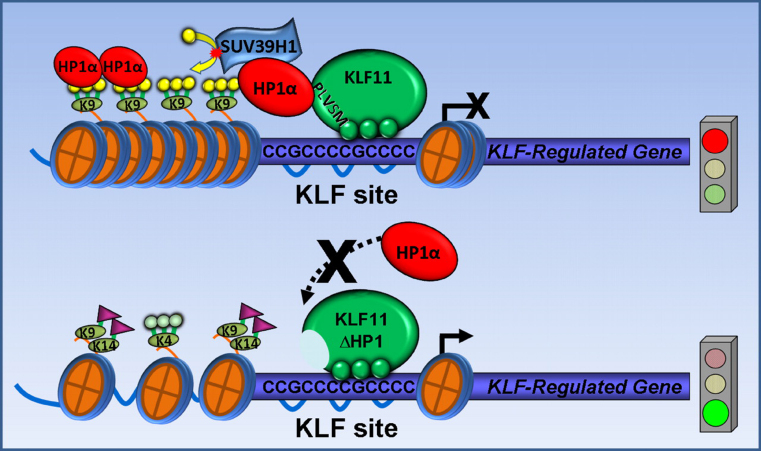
Model of HP1 recruitment in KLF11-mediated gene regulation. An extended KLF11 binding site mediates the sequence-specific recruitment of HP1-SUV39H1 to regulate gene promoters (*upper*). Our experimental data demonstrate that engagement of HP1-SUV39H1 recruitment by KLF11 binding leads to the deposition and/or removal of key histone marks that change the state of chromatin and impact on transcription. Disruption of this recruitment mechanism, as achieved by KLF11ΔHP1, leads to derepression of gene promoters (*lower*).
